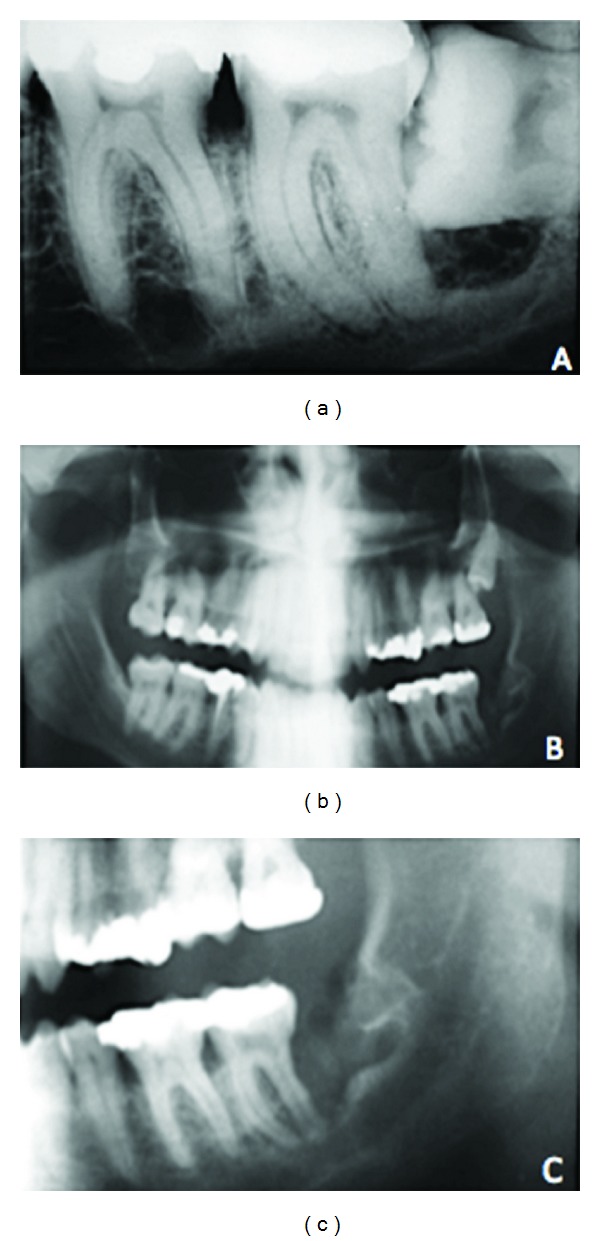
(a) Periapical X-ray showing a radiolucency surrounding the crown of the left third molar suggestive of dentigerous cyst. (b-c) Panoramic X-ray performed after 15 days of the third molar extraction showing no suggestive malignant transformation.
